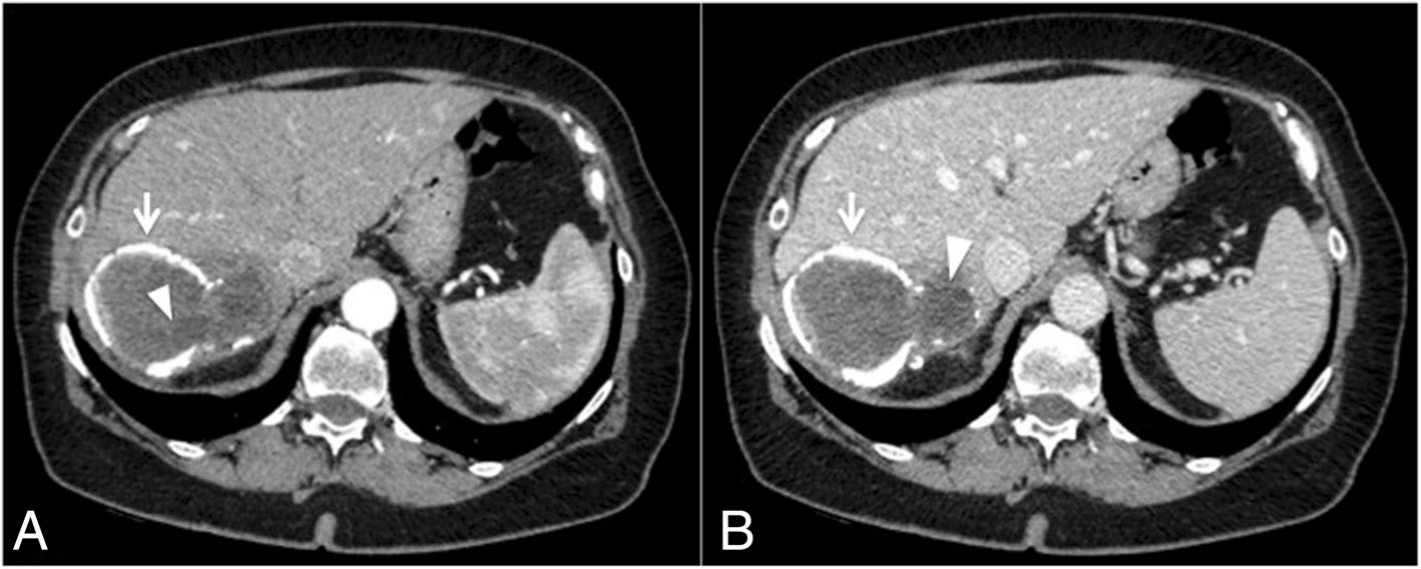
Axial CT view after intravenous contrast media administration during arterial (a) and venous (b) phase, shows a big cystic lesion of the liver, at segment VII, with subtotally calcified walls (arrows) and internal daughter cysts (arrow-head)
